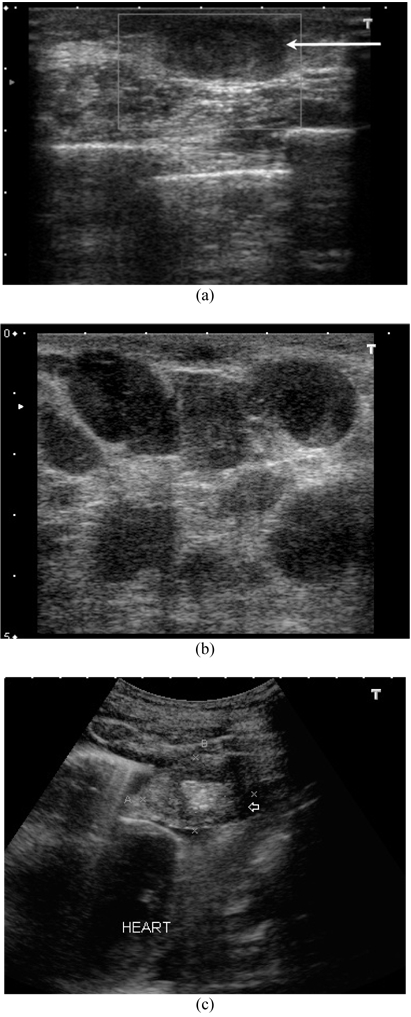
a) Ultrasound, transverse section through the palpable lump. Note the hypoechoic nodule (arrow) with micro lobulation. The Doppler colour box shows no blood flow signals; b) Ultrasound of the left axilla. Note cluster of enlarged nodes; these are round and have lost the central echogenic hilum suggesting malignant infiltration; c) Ultrasound through a left intercostal space, showing a parietal mass in the left pleural cavity (arrow) that touched the heart on real-time scanning.
